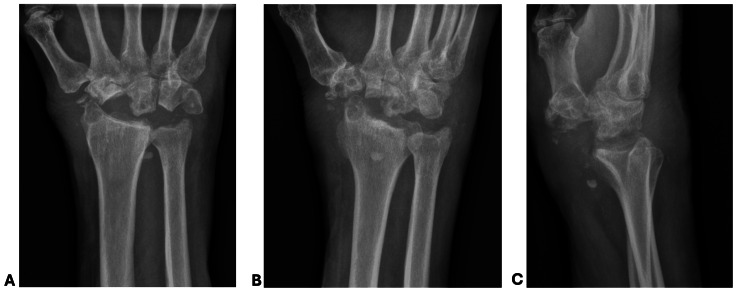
Postoperative radiographs of the right wrist at four weeks AP: anteroposterior Right wrist X-rays including AP (A), oblique (B), and lateral (C) projections with postoperative changes following proximal row carpectomy. No radiographic evidence of progressive erosive changes in the remaining carpal bones
